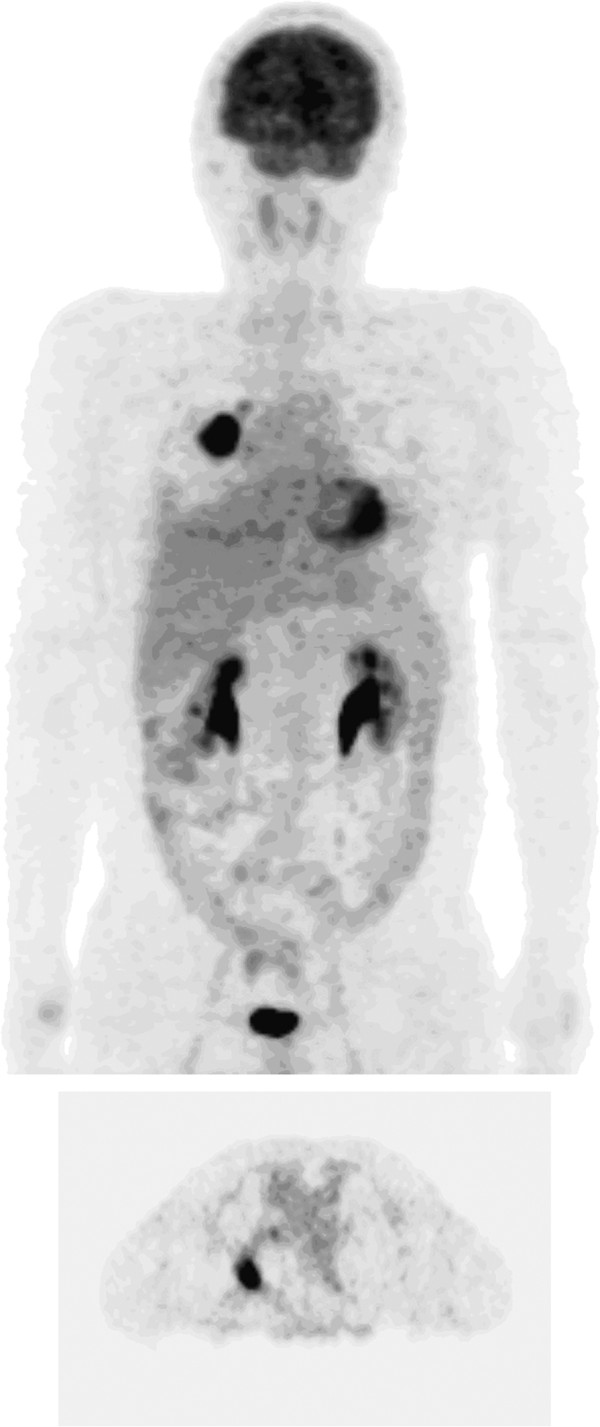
An 18F-fluorodeoxyglucose (FDG) positron electron tomography (PET) scan showing lesions with obviously increased standardized uptake values (SUVs) in the lower lobe and hilum of the right lung.
